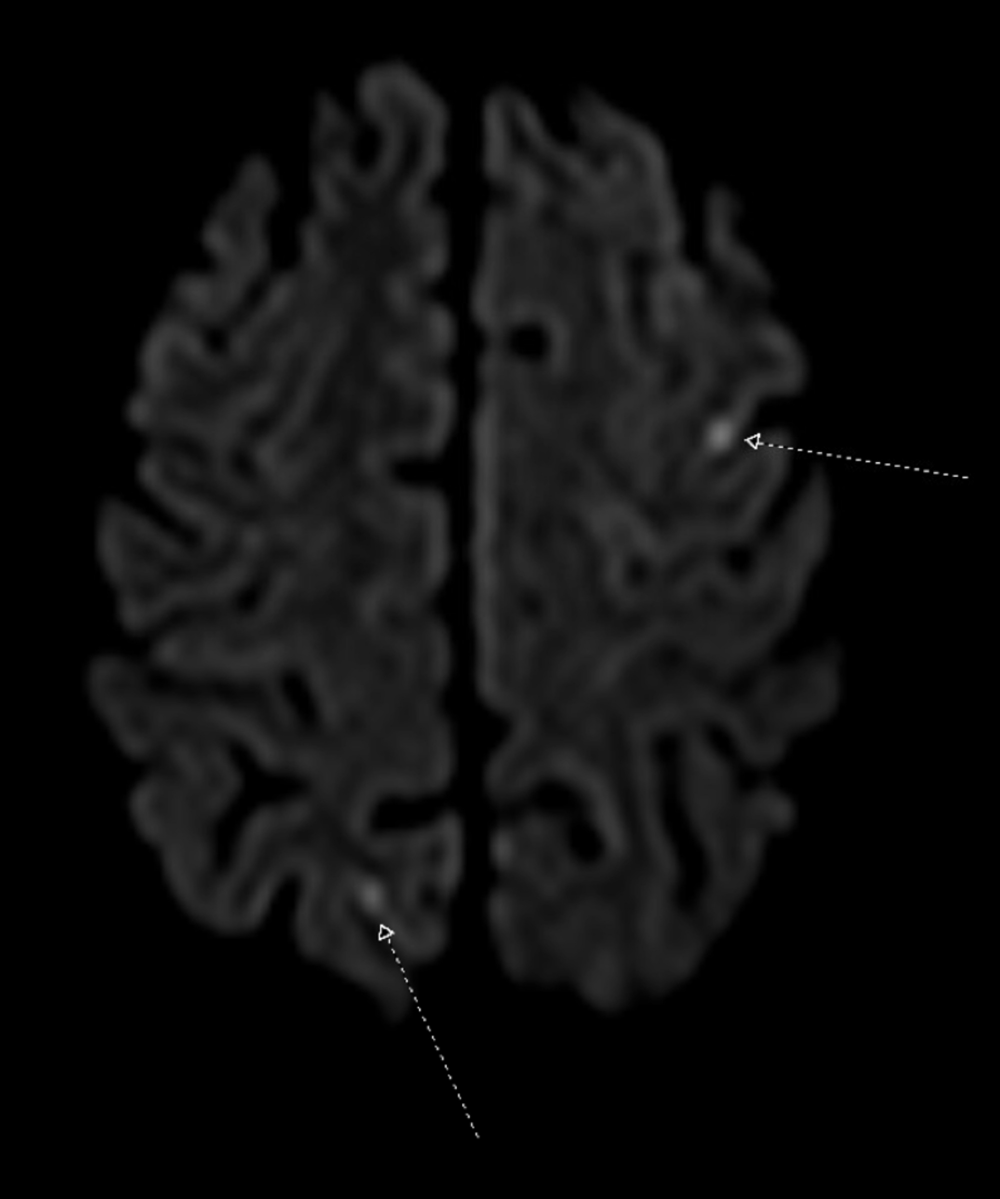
Magnetic Resonance Imaging Brain Noncontrast This image demonstrates two regions of focal restricted diffusion in the right parietal lobe and the left temporal lobe, which represent acute embolic infarcts.
